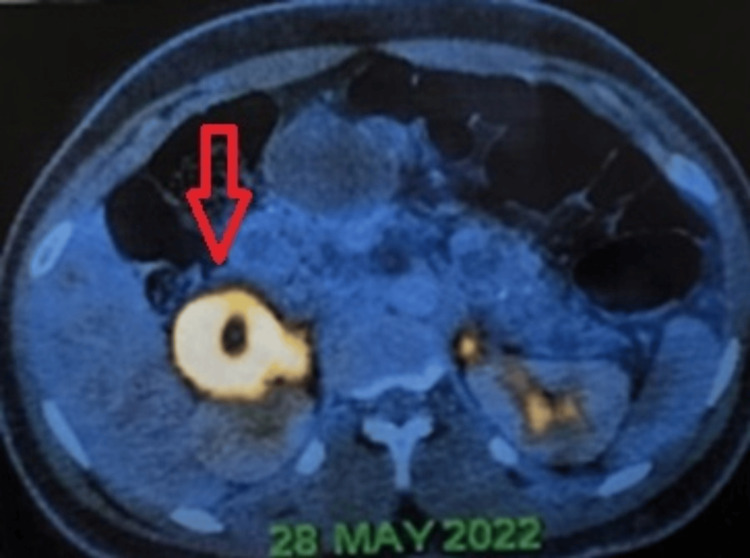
Positron emission tomography-computed tomography image showing a lesion with increased metabolic activity in the right suprarenal fossa.
